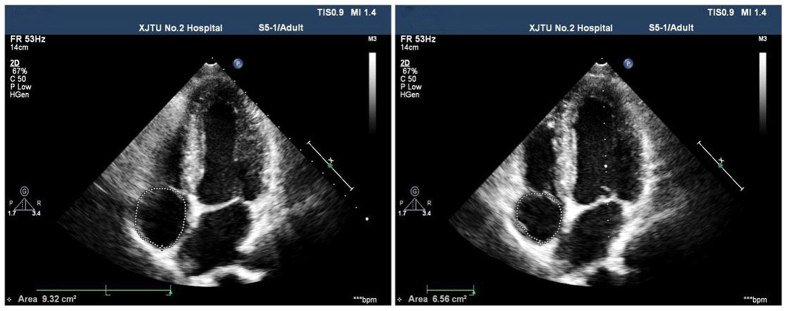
Measurement of the left ventricular end-diastolic volume and area using the modified Simpson’s rule in apical 4-chamber views at left ventricular end-diastole at rest and during the Valsalva maneuver.
